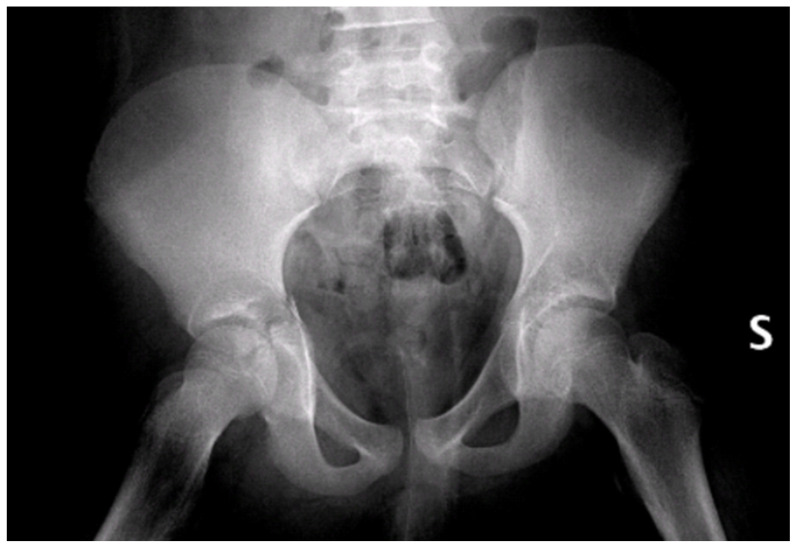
Pelvic X-rays at admission time. S = left side.
